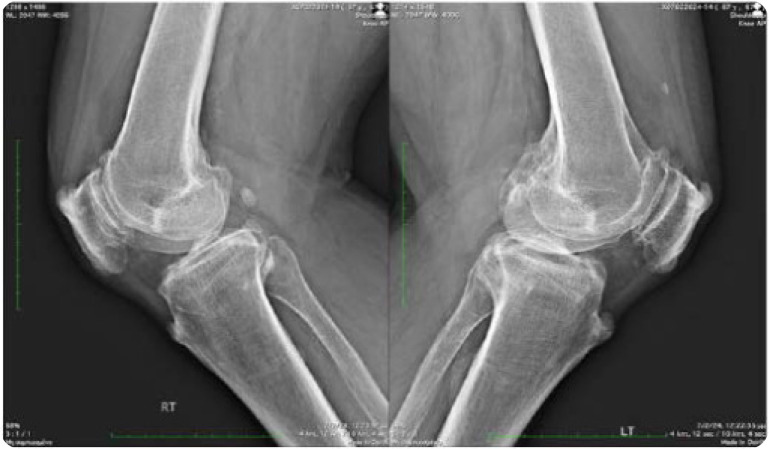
Lateral view of knees x-rays shows calcification and ossification of the superior and inferior patellar poles as well as ossified tibial tuberosities with enthesopathy bilaterally.
